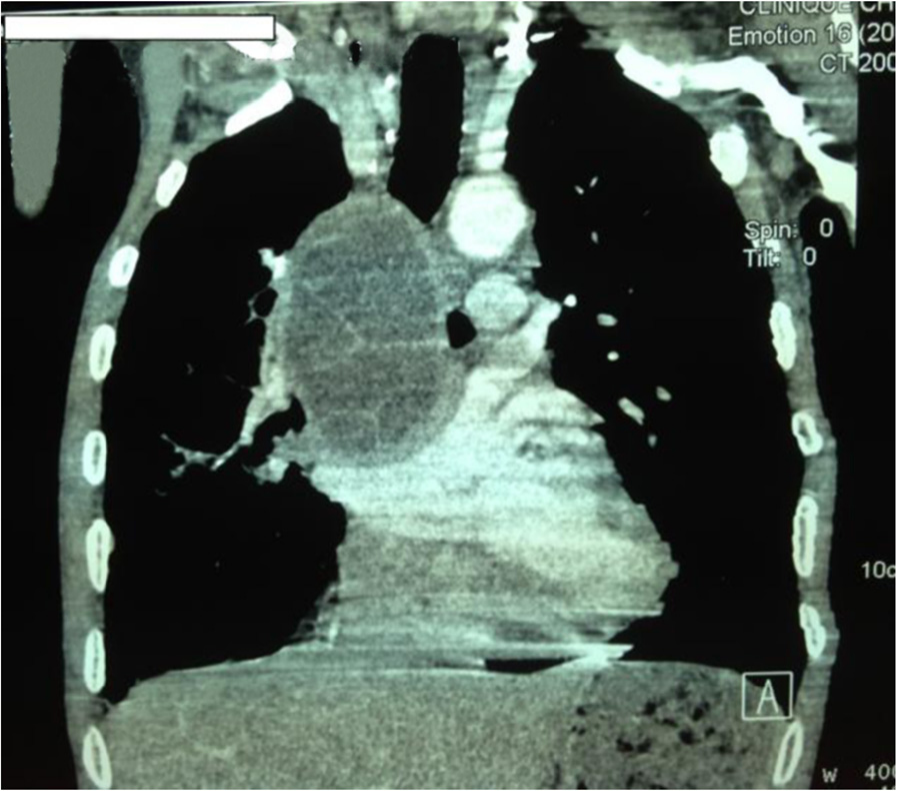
Computed tomography image reconstruction showing the close relationship between the cyst and the bronchus.
